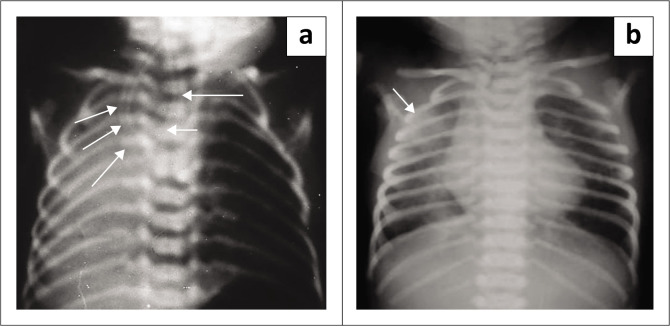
(a) Frontal radiograph of a neonate depicting the coiled nasogastric tube (white arrows), multiple vertebral anomalies, and an opaque right hemithorax with ipsilateral mediastinal shift suggestive of lung hypoplasia. (b) Another neonate in whom there was difficulty in passing the nasogastric tube showed aspiration-related changes in the right upper lobe (white arrow).
